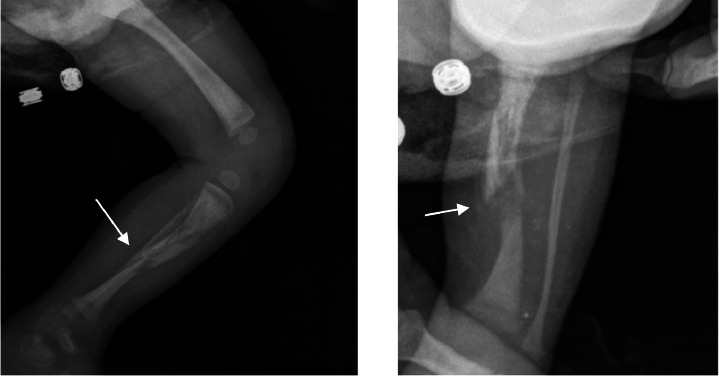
X-ray of the left lower limb identifies a mid-diaphyseal tibial fracture (white arrow).
